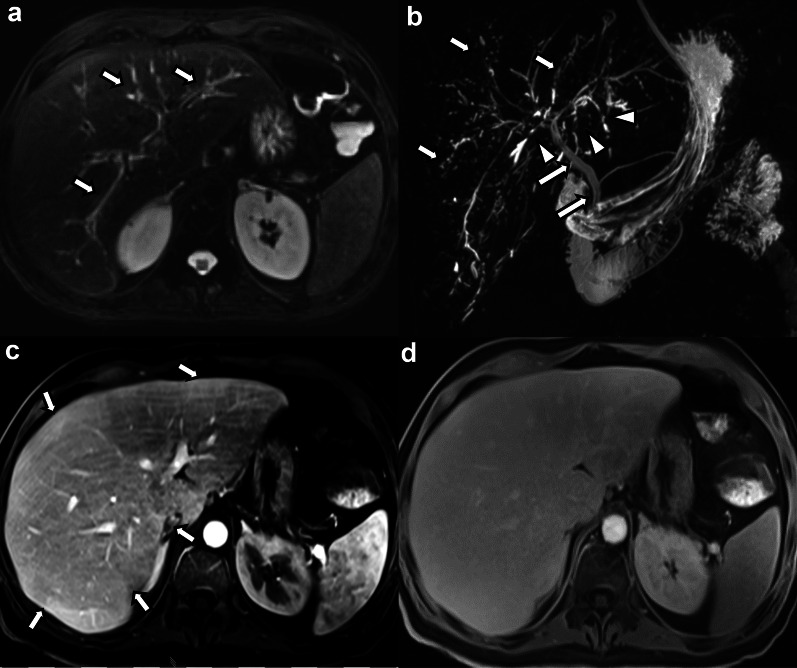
40-year-old male patient with COVID-19-associated SSC after severe COVID-19 infection with ARDS and ECMO requirement. a Axial fat-suppressed T2-weighted image shows mildly accentuated intrahepatic bile ducts with hyperintense peribiliary signal changes (arrows). b Maximum intensity projection MRCP image shows multifocal beading of the intrahepatic bile ducts (arrows) and multiple short-segment strictures (arrowheads). The extrahepatic biliary tree is spared (long arrows). c Post-contrast subtraction image in the arterial phase shows subtle inhomogeneous parenchymal enhancement with areas of hyperenhancement (arrows). d Hepatobiliary phase image acquired 20 min. after intravenous administration of gadoxetate disodium shows decreased hepatobiliary uptake with decreased liver-to-vessel-contrast. The patient underwent orthotopic liver transplantation and explant pathology revealed findings consistent with a severe ischemic cholangiopathy and grade F2 fibrosis
